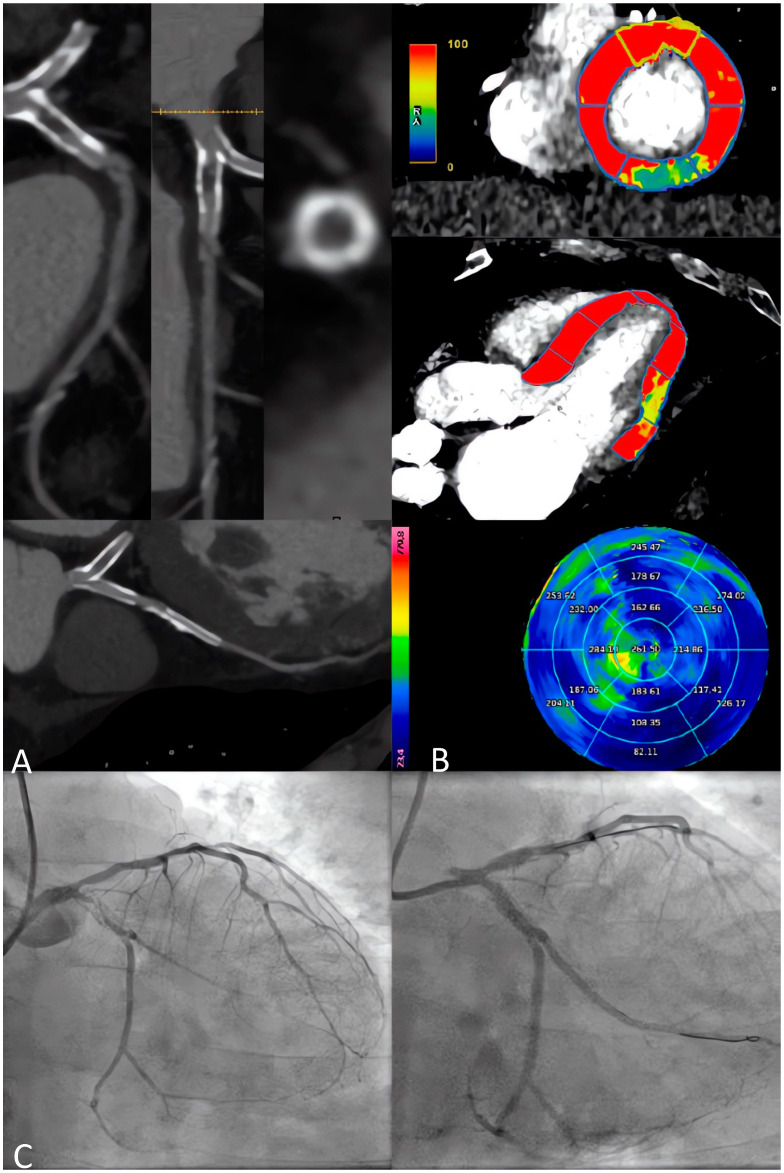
A 65-year-old male patient with a previous history of PCI on LAD and LCx, complaining of the recurrence of effort angina. (A) CCTA shows sub-occlusive intrastent re-stenosis of LCx, while the LAD stent appears with some intimal hyperplasia but no severe restenosis. (B) The s-CTP with dynamic quantitative perfusion shows an inducible hypoperfusion defect on the inferior-lateral basal segment. (C) ICA confirms intrastent sub-occlusive restenosis of LCx treated by PCI, while LAD shows non-severe intrastent restenosis, unworthy of PCI. CCTA: Coronary Computed Tomography Angiography; ICA: Invasive Coronary Angiography; LAD: Left Anterior Descending; LCx: Left Circumflex Artery; PCI: Percutaneous Coronary Intervention.
